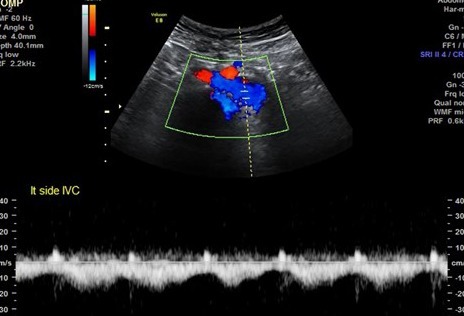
Pulsed Doppler ultrasonography image at the epigastric region showing a
